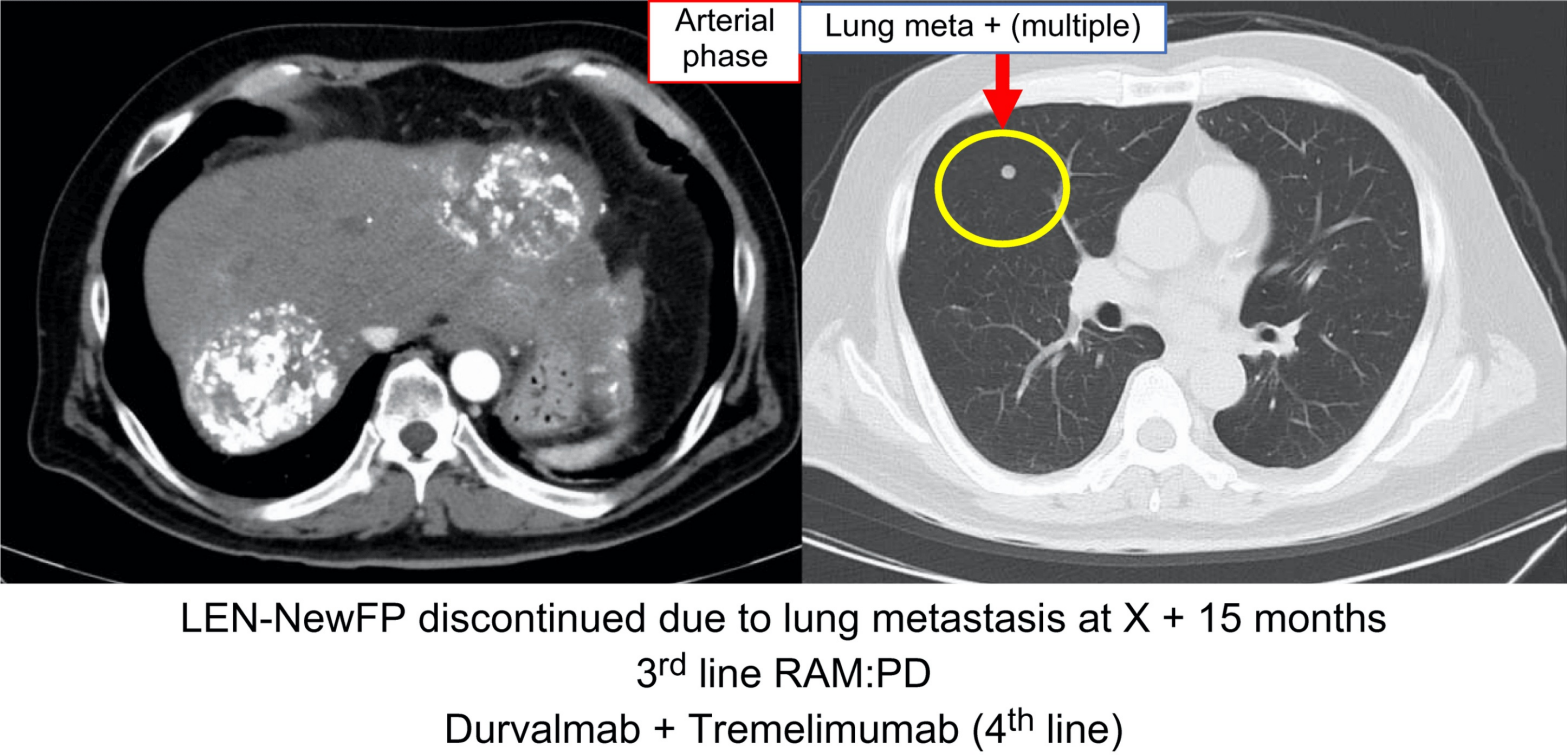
Contrast-enhanced CT on X+2 February showed good intrahepatic disease control and no intrahepatic viable tumor; however, a new pulmonary metastasis was detected. LEN: lenvatinib; RAM: ramucirumab; PD: progressive disease
